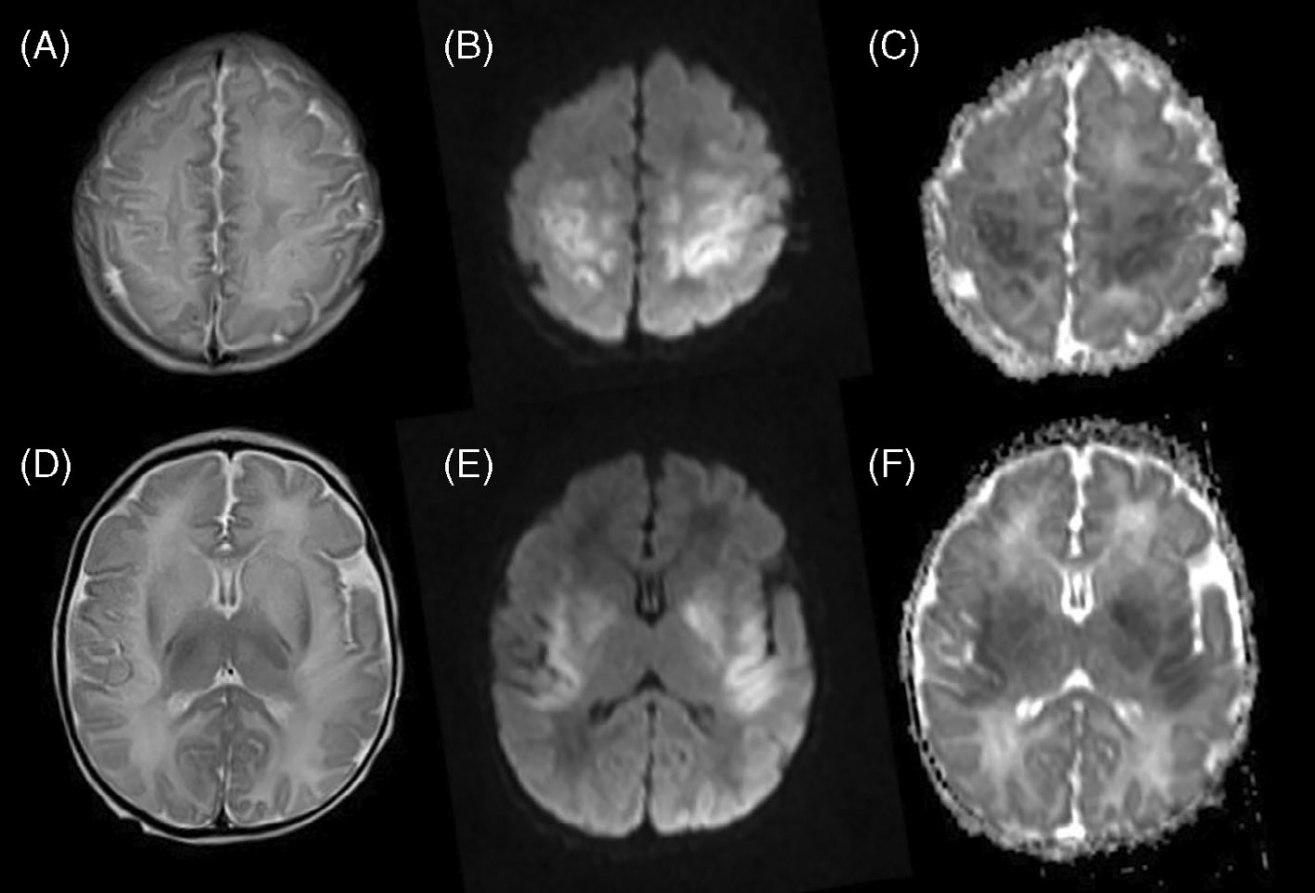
MRI of patient 2 at the age of 9 days: (A and D) axial T2‐weighted images, (B and E) diffusion weighted images, and (C and F) ADC maps show bilateral symmetric faint abnormal T2 signal intensity and diffusion restriction in the perirolandic cortex (images A–C) and in the insula and basal ganglia (images D–F).
